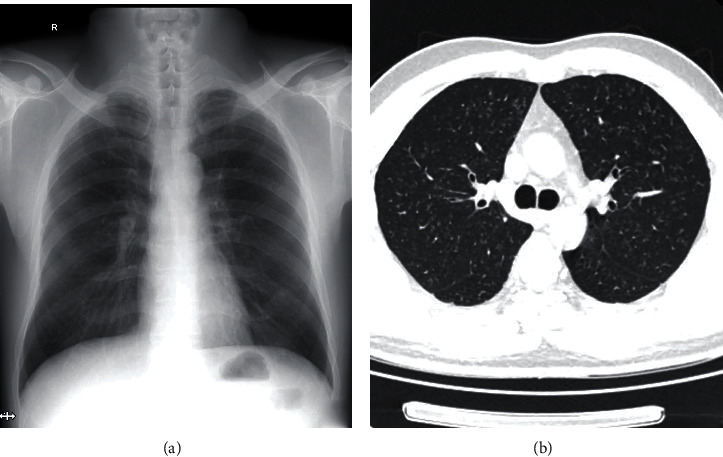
Typical case from a patient with stage I of occupational silicosis. (a) High KV DR show increased and disordered lung markings; small dot shadow is not obvious. (b) CT shows diffuse miliary nodules in both lungs with ground glass-like changes.
